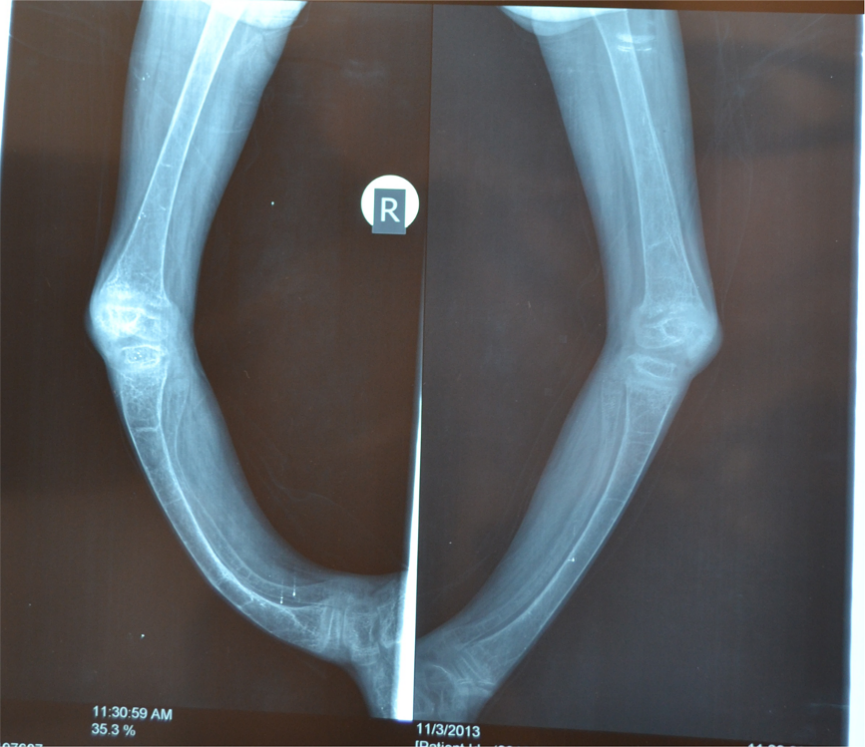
X-rays of both lower limbs showing severe bowing of the legs and diffuse osteopenia. It also shows dense transverse lines in the tibia suggestive of looser’s zones indicative of rickets.
